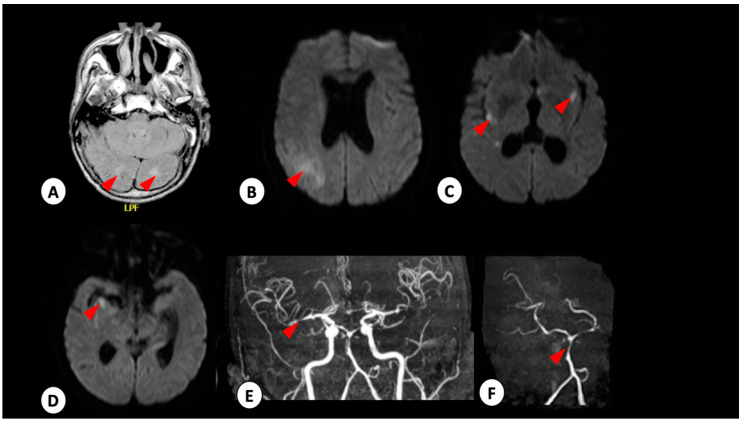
Patient 3 radiologic observations. Brain NMR performed 48 h after admission. Red arrowhead shows ischemic infarct in cerebellum (A), cerebellum (B), base nuclei (C) and hippocampus (D) in FLAIR (A) and diffusion-weighted image (B–D). (E,F) Stenosis in medium cerebral artery and stenosis in basilar artery, respectively.
